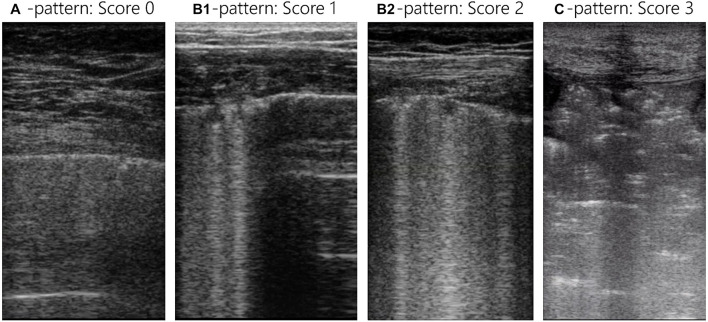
Lung ultrasound images for all LUS patterns and scores. Each LUS image was scored with the LUS aeration score: an “A-pattern” (i.e., repeating horizontal A-lines parallel to the pleural line) was scored “0,” a “B-pattern” (i.e., ≥3 vertical B-lines starting from the pleural line and reaching the bottom of the screen) was scored “1” if B-lines are well-spaced and cover ≤50% of the pleural line, and “2” if B-lines cover ≥50% of the pleural line, and a “C-pattern” (i.e., consolidation) was scored “3.” The global LUS score is the sum of all 12 lung regions and reaches from 0 to 36 and the anterior, lateral, and posterior LUS score are the sum of 4 lung regions and reach from 0 to 12. LUS, lung ultrasound.
